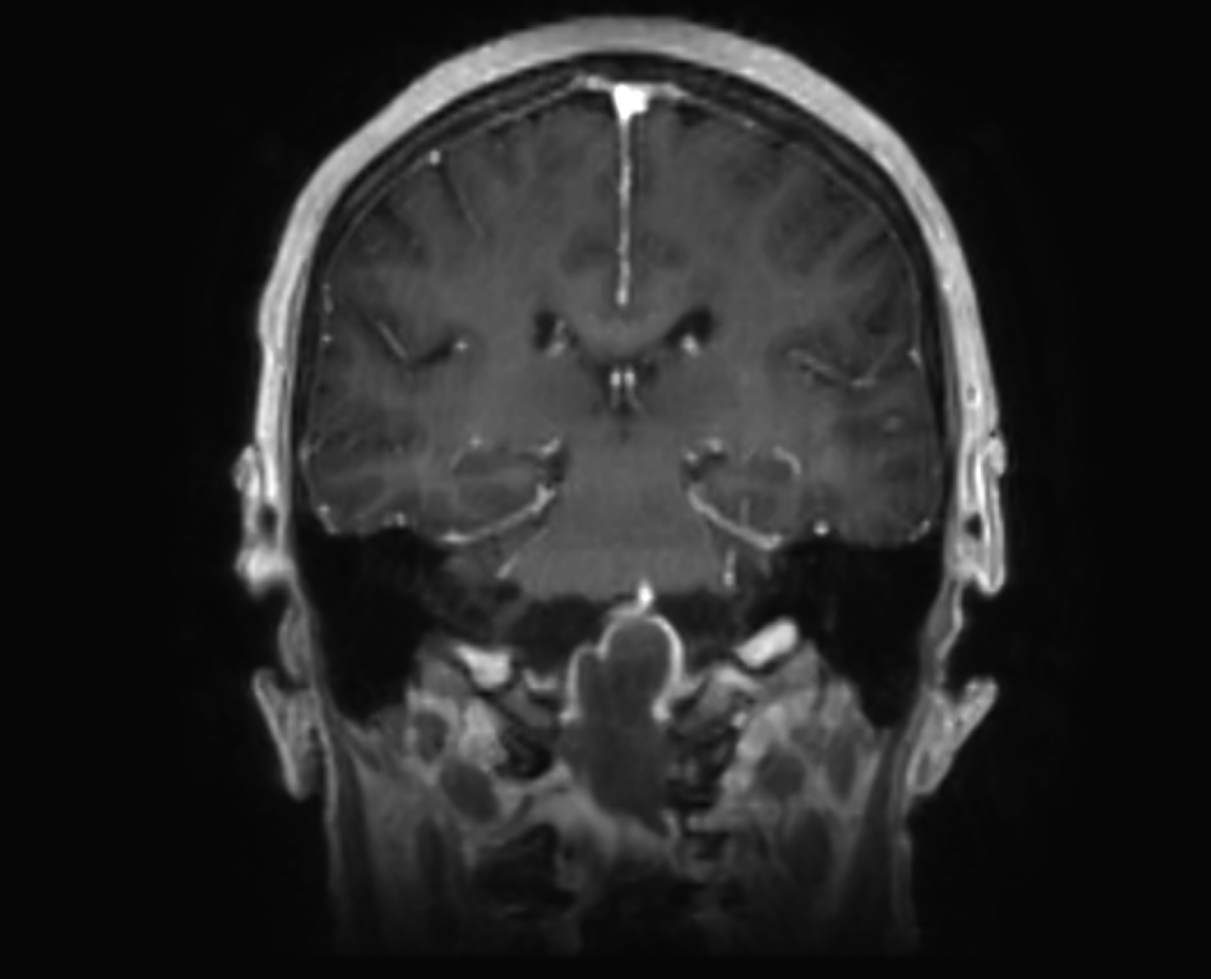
February Coronal MRI Craniocervical junction mass
